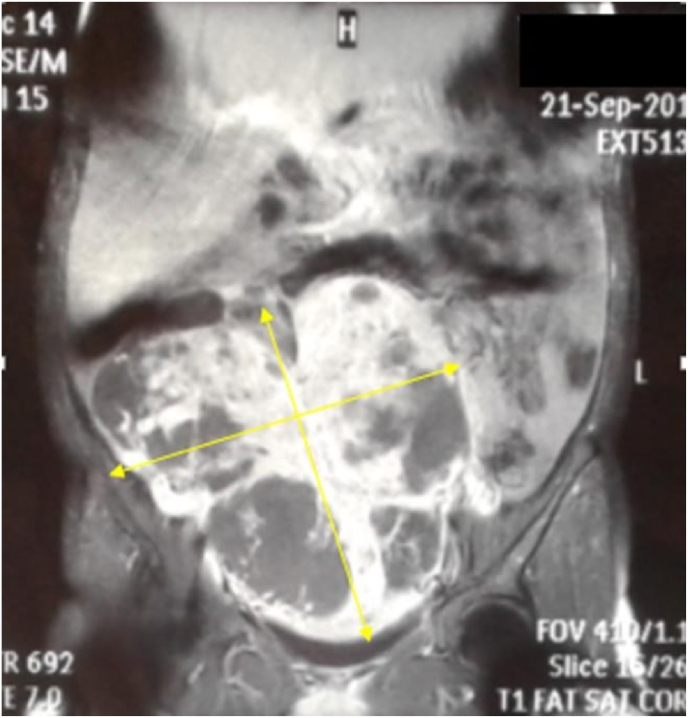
Pelvic MRI in coronal slices and T1 sequences showing a large solid cystic abdominal mass in T1 heterosignal, heterogeneously enhanced after gadolinium injection, measuring 220x100 × 175mm. This mass is poly-lobed and comes into contact with the uterus, the rectum and the bladder, with preservation of a separation line.
